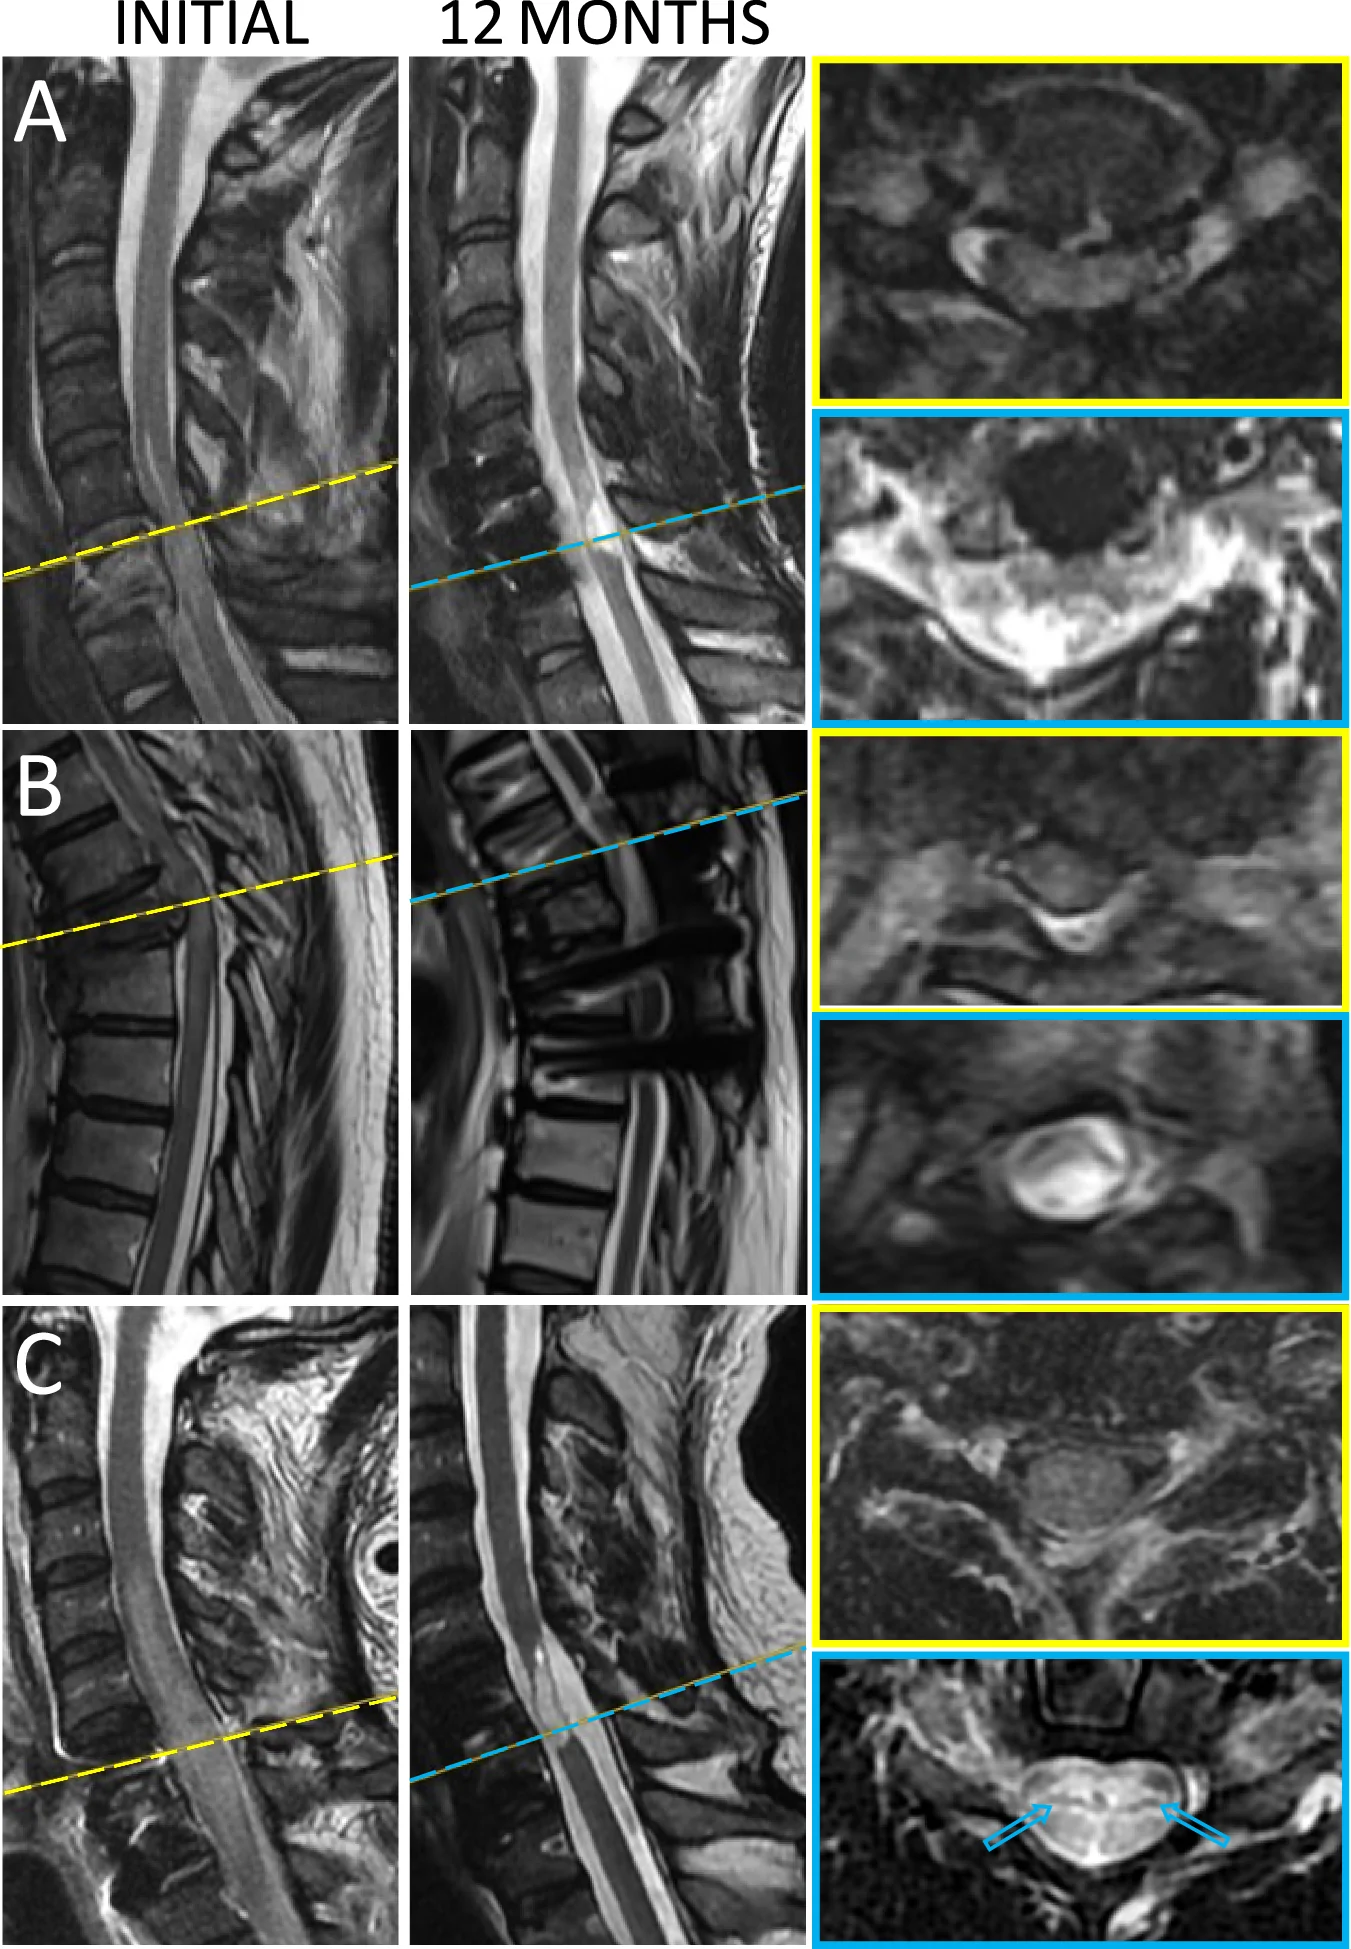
Initial and final MRI images. A T2-weighted sagittal and axial sequences of participant 2 obtained before and 12 months after polylaminin injection. Initial images show a fractured C6 vertebra and extensive oedema throughout the spinal cord cross-section. At 12 months, an oval-shaped hyperintense area consistent with fluid occupied the lesion site. B T2-weighted sagittal and axial sequences of participant 4 before and 12 months after treatment. Final MRI images were affected by prosthesis-related artifacts; however, findings suggest the formation of a cystic cavity at the lesion site. C T2-weighted sagittal and axial MRI sequences of participant 6 before and at 12 months after treatment. Initial images reveal extensive oedema across the entire spinal cord cross-section, with interruption of cerebrospinal fluid flow. Final images show marked hyperintensity at the lesion site, adjacent to a narrow strip of preserved tissue (arrows in axial view).
